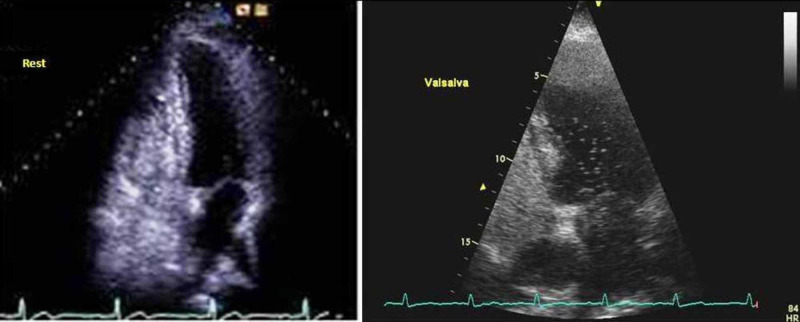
Bubble contrast transthorachic echocardiography a) on rest, b) during Valsava maneuver
